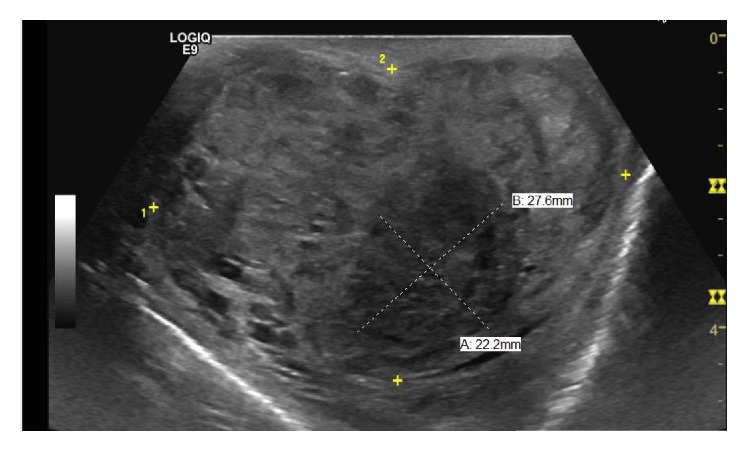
Ultrasound of the L testicle, revealing a heterogeneous infiltrating solid mass.
